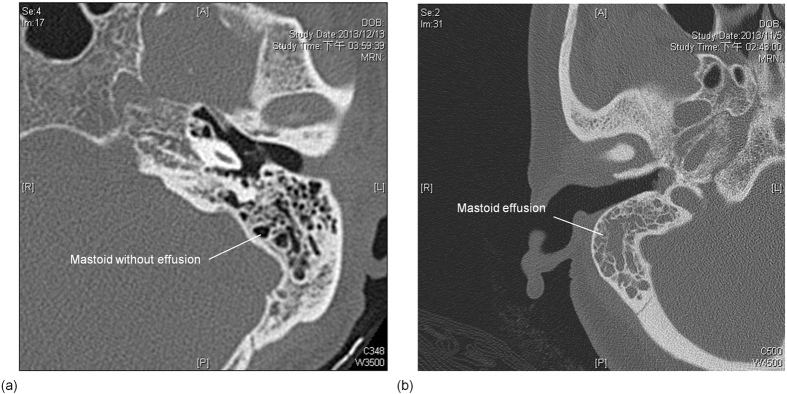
Typical computed tomography images of mastoids for patients without (left) and with MEE (right) captured at Chang Gung Memorial Hospital at Linkou, Taiwan. Air cells in the mastoid correspond to gray shading, representing a water-based medium (effusion).
